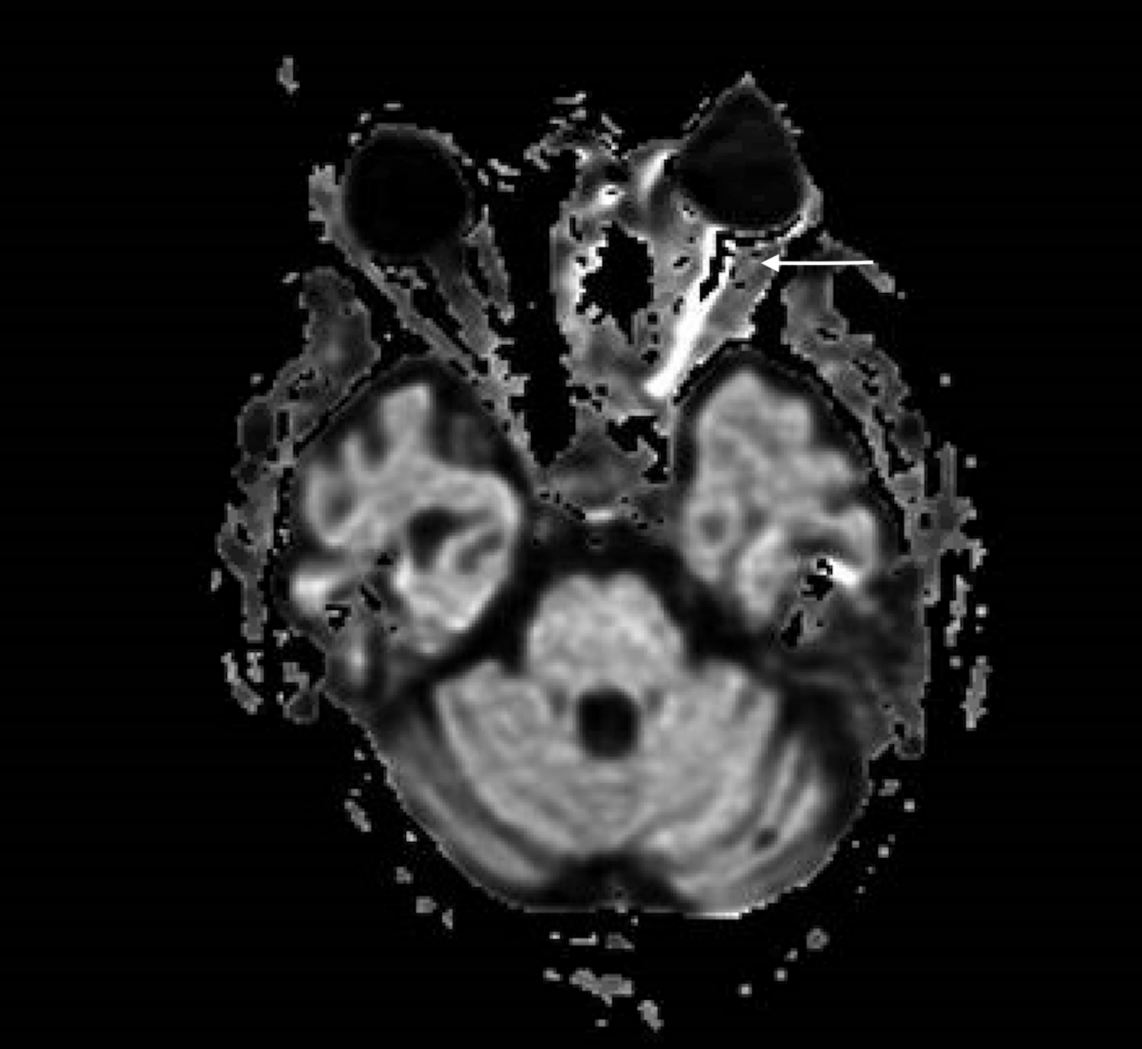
Magnetic resonance imaging of the head, orbit, face, and neck with contrast showing optic nerve inflammation/ ischemia.
